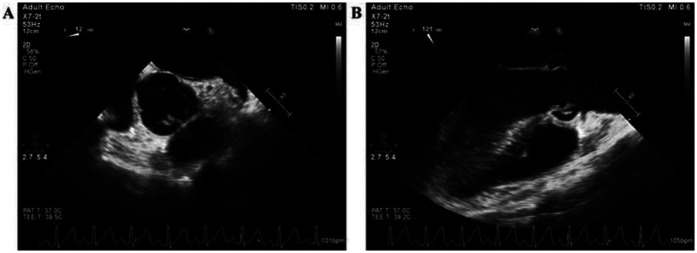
The image of transesophageal echocardiography before anti-infective therapy.
